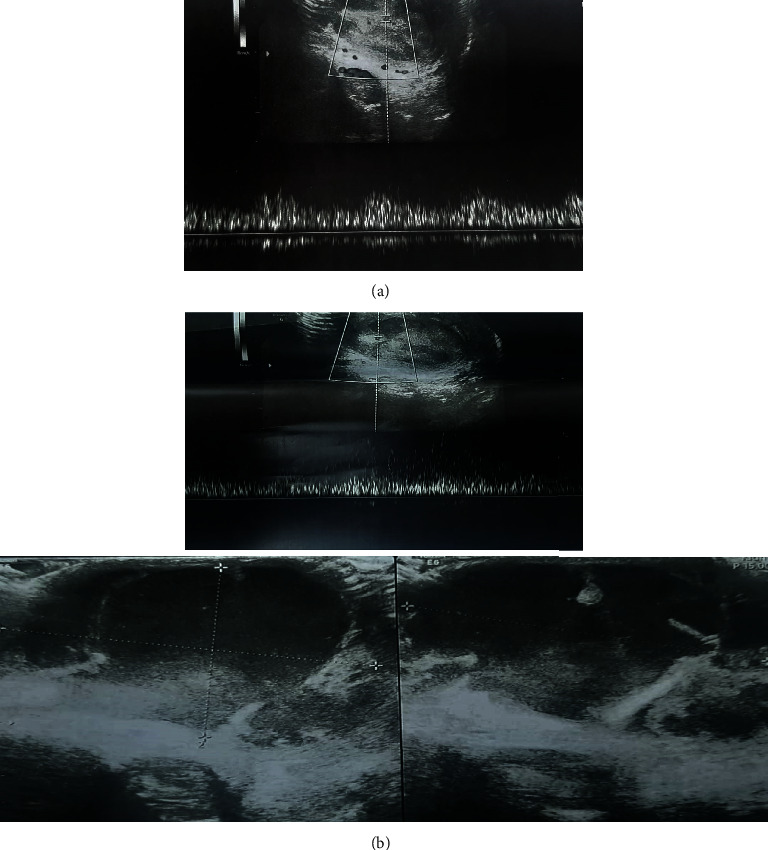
(a) Chest CT and (b) Sonography report.
